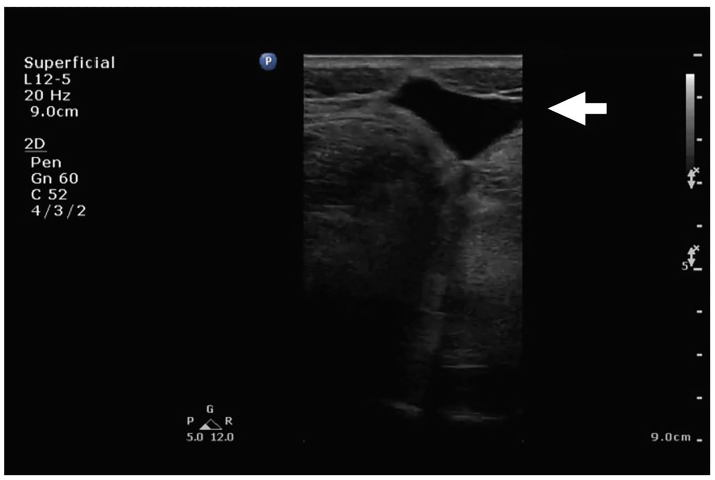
Sagittal ultrasound image of a simple fluid collection (arrow) tracking from the skin to the spine.
